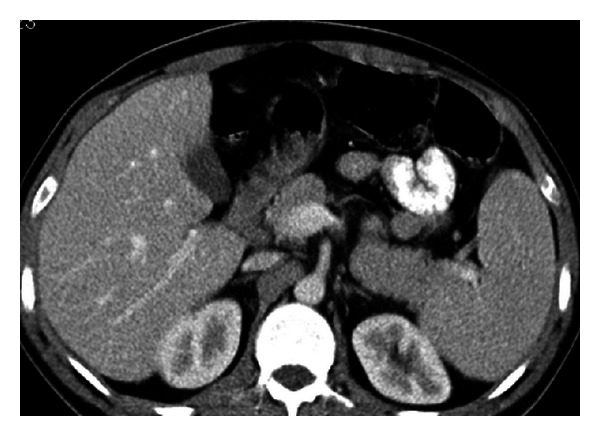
Axial contrast enhanced CT image of a 29-year-old male patient shows splenopancreatic fusion anomaly.
